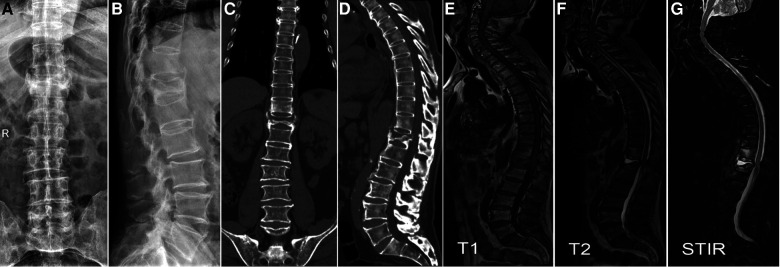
A 66-year-old male patient with T11 and T12 fractures (A,B). Coronal (C) and sagittal (D) CT showed intravertebral vacuum phenomenon (IVP). MRI showed well-defined hypointensity on T1 (E), hyperintensity on T2 (F), and hyperintensity on short time inversion recovery (G), verifying the diagnosis of IVP.
